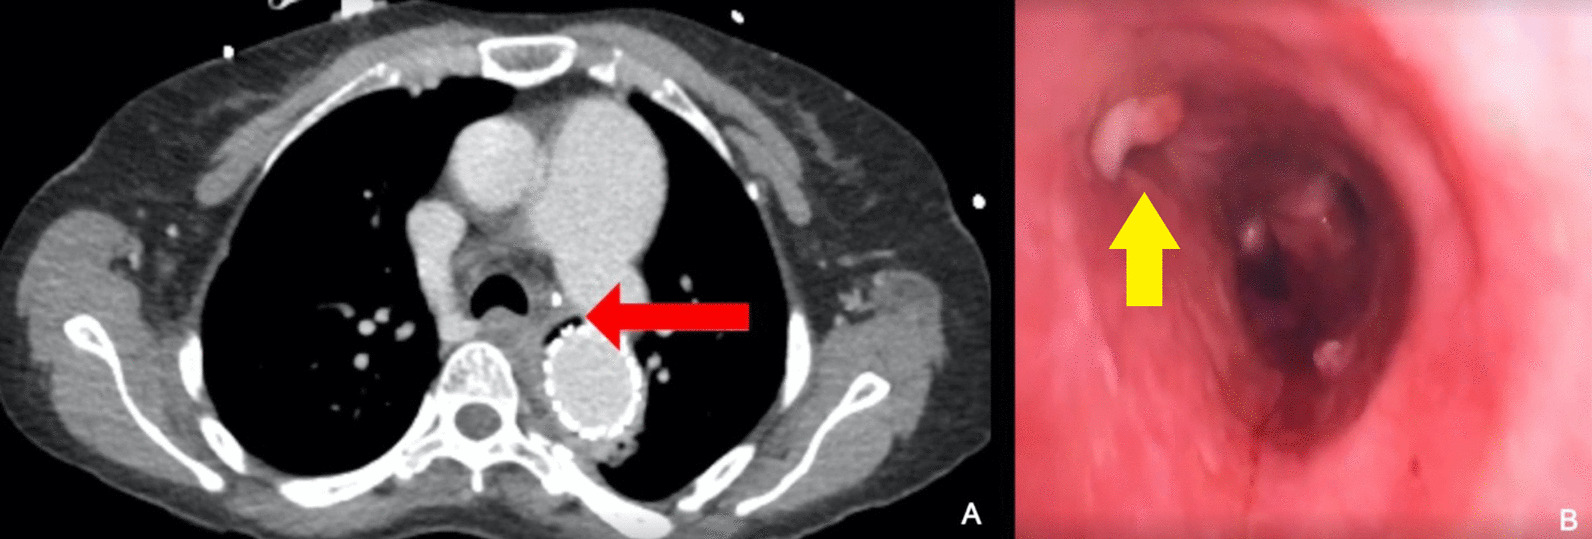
A CT image demonstrating air adjacent to the endograft within the descending thoracic aorta (red arrow). B A defect in the wall of the left main bronchus with fibrino-purulent exudate is visible at flexible bronchoscopy (yellow arrow)
